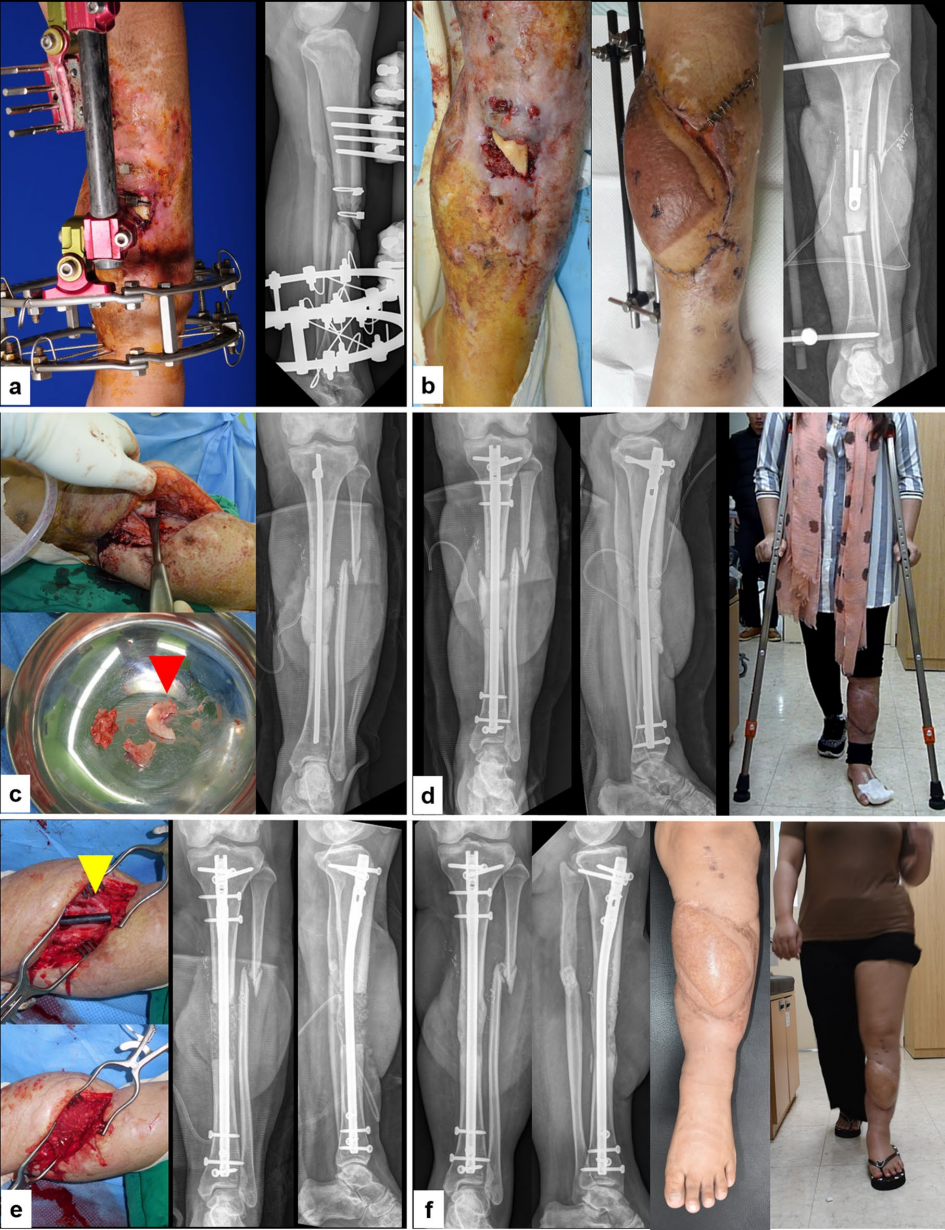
(a) A 24-year-old female patient sustained a recalcitrant infected nonunion of the left tibia. (b) According to the Multi-staged IMT protocol, we performed thorough debridement, placed an antibiotic-loaded PMMA spacer, and provided temporary bony stabilization. Soft tissue defects were covered using an anterolateral thigh flap (stage 1). (c) As PDCP had been identified at EMP and IMP (pseudomonas and MRSA), targeted debridement at proximal area of bone defect (red arrow head, sequestrum) and temporary fixation using antibiotics loaded PMMA coated intramedullary rod was performed (repetitive stage1). (d) After confirming all PDCN at previous stage, definitive fixation using IM nail was performed. An antibiotic-loaded PMMA spacer was reinserted into the bone defect for staged bone grafting to confirm that the infection had been eradicated (stage 2). Prompt partial weight bearing exercise was allowed after definitive fixation. (e) The 6-cm sized segmental bone defect was filled with autogenous bone (45 g) and DBM (15 g) in induced membrane chamber (yellow arrow head) at 16 weeks after stage 2 (stage 3). (f) Two years follow up radiographs show complete consolidation of the grafted bone without recurrent infection. The injured limb recovered to full function.
